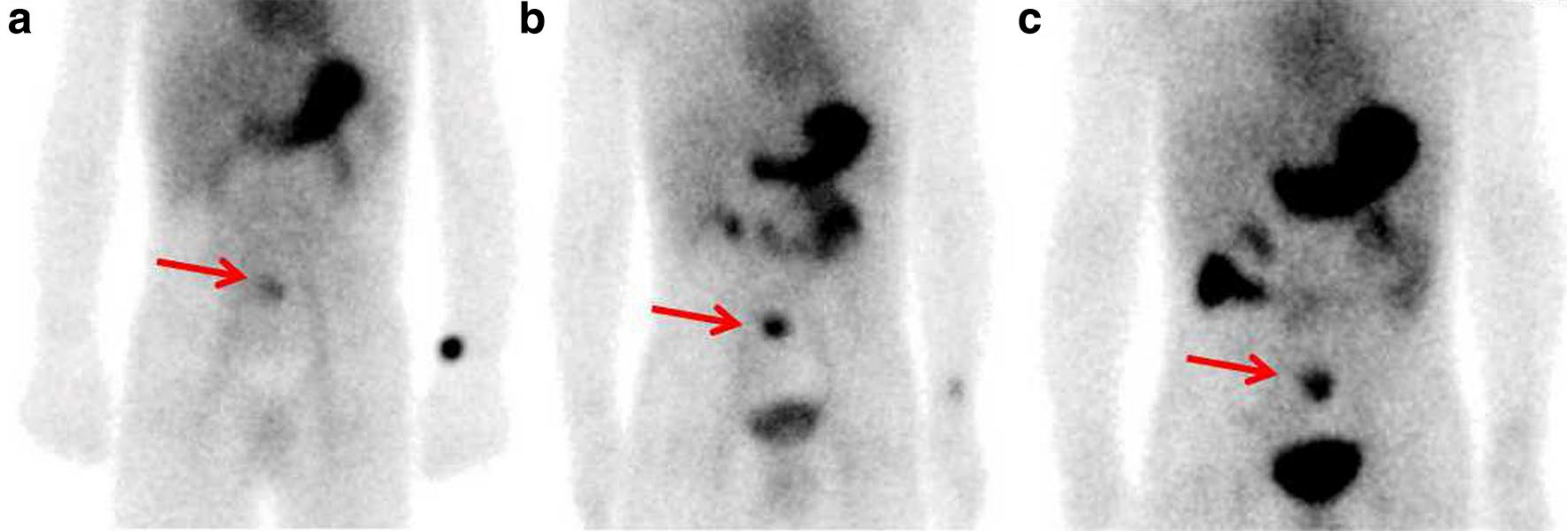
5 min (a), 20 min (b) and 60 min (c) after administering 1 mCi of Technetium-99 m pertechnetate intravenously, planar scintigraphy was performed respectively. Images showed a focal concentration at the small intestine region of the lower abdomen
